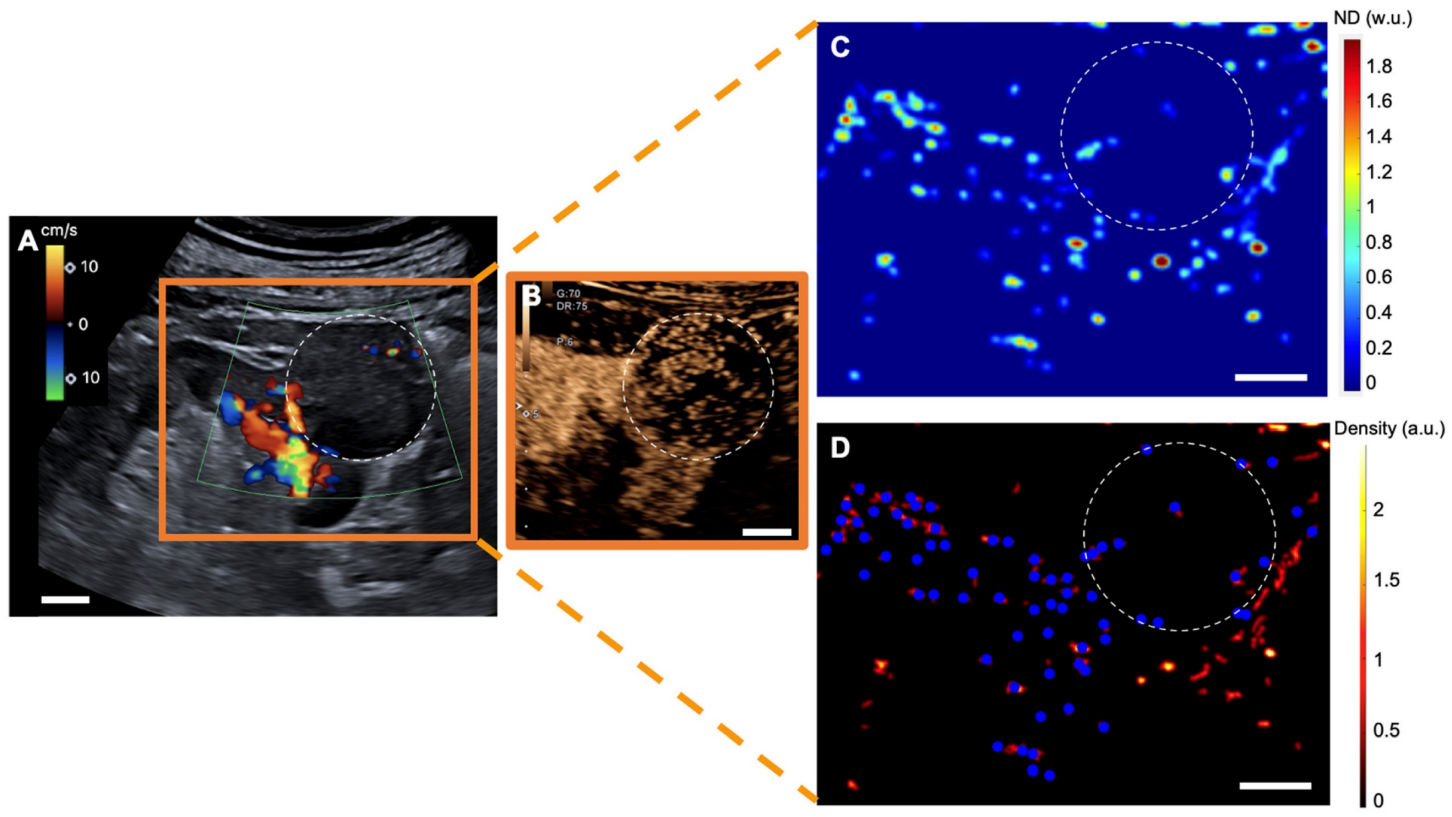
sULM of clear cell renal cell carcinoma (ccRCC) (patient 1). A & B. US Doppler image (A) and CEUS (B) showing an exophytic renal tumor (ccRCC) (*white-dotted area*). C & D show normalized distance metrics. (C) This metric enhances glomerular behavior, highlighting the detected glomeruli in blue points on the density map; (D) Note the presence of glomerular paths in the renal cortex and some artifacts mimicking glomeruli in the renal tumor. (The traces projected on the grid are displayed in red on the image. The colorbar corresponds to the count of the number of bubbles per pixel. Scale bars indicate 10 mm. *ND= normalized distance; w.u.= without units; a.u. = arbitrary units*.
